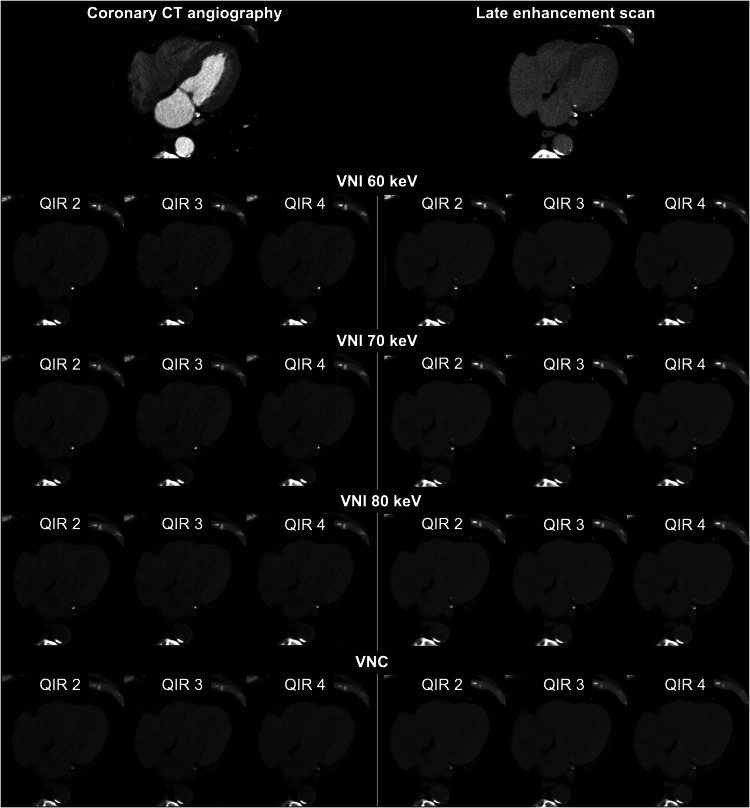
Representative CT images obtained in an 85-year-old man with severe aortic stenosis examined for transcatheter aortic valve replacement planning. Axial CT images acquired with dual-source photon-counting detector CT show the virtual non-iodine (VNI) images at 60, 70, and 80 keV and the virtual non-contrast (VNC) images reconstructed from coronary CT angiography and cardiac late enhancement scan with quantum iterative reconstruction (QIR) strengths 2, 3, and 4
